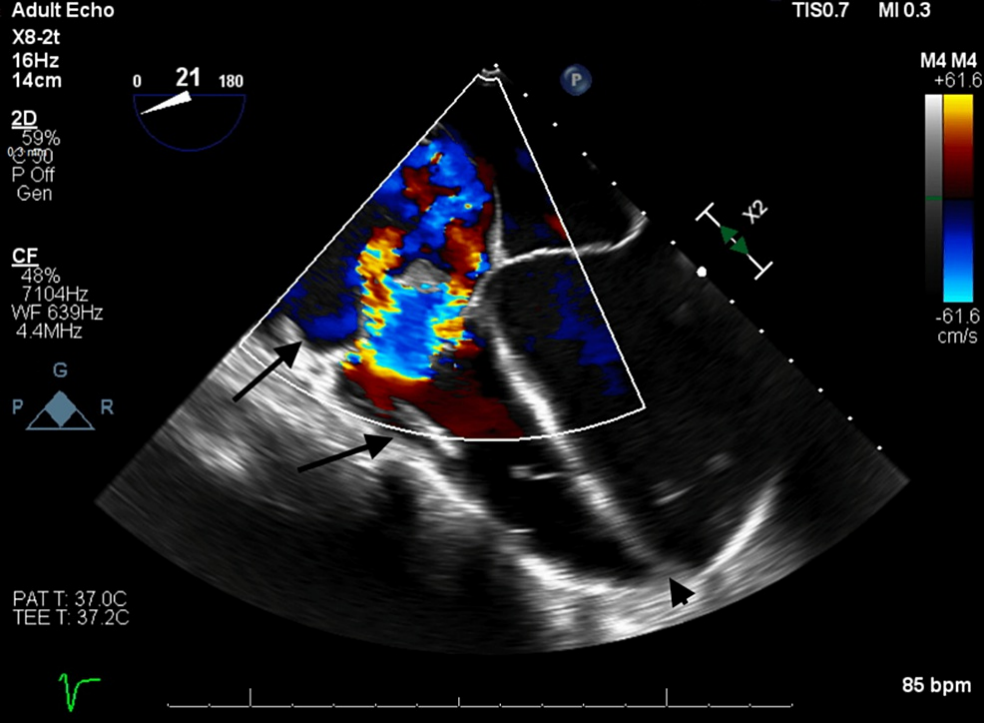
TTE showing torrential tricuspid regurgitation (colored), right atrial and ventricular dilation (arrows), and flattening of the intraventricular septum (arrowhead). TEE, transesophageal echocardiogram
